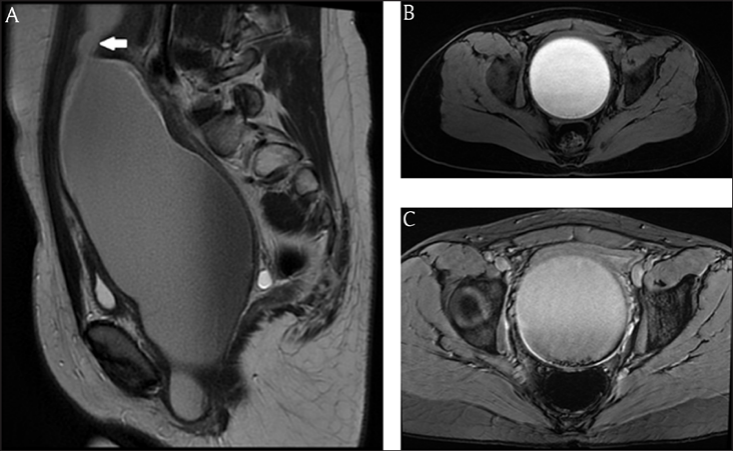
MRI: A. Sagittal T2-weighted image showing a large cystic mass with T2 hyper- to iso-intense content. Arrow = uterine cervix. B. Axial T1-weighted image showing a large cystic mass with T1 hyperintense content. C. Axial T2*-weighted image showing susceptibility artifacts posteriorly in the lesion.
